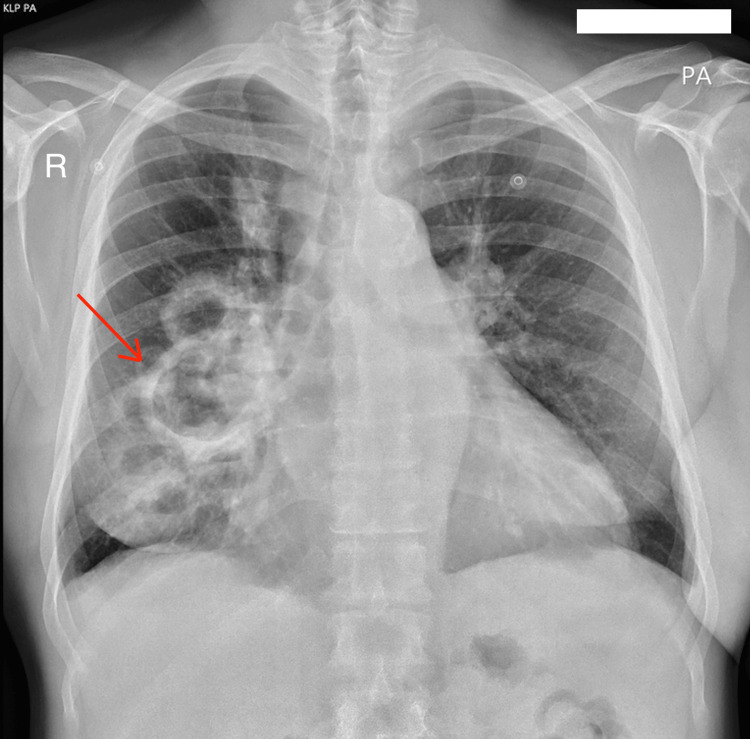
Chest X-ray on admission with visible lesion in right lung (red arrow).
